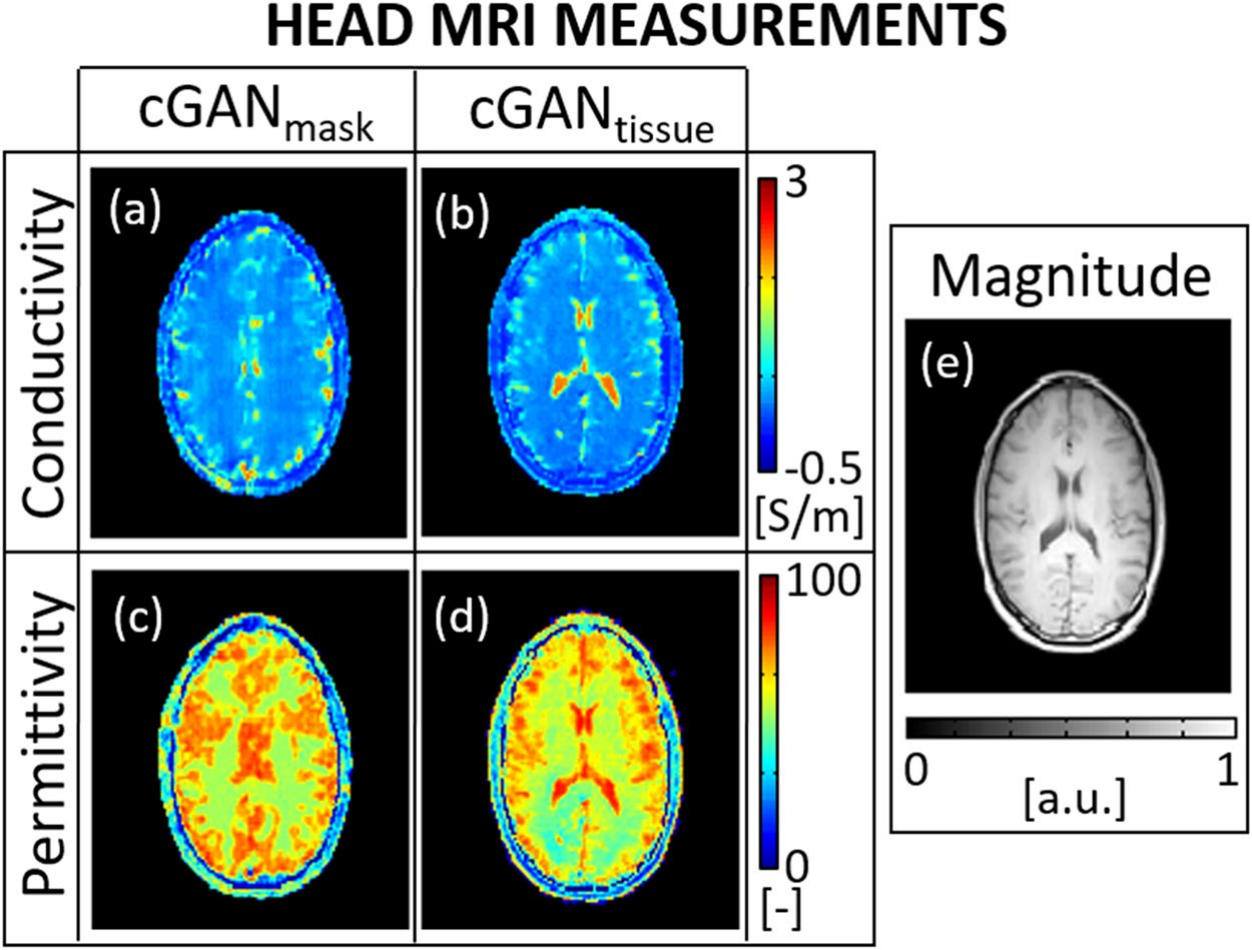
DL-EPT conductivity and permittivity reconstructions from MR measurements on the first subject using cGAN_mask_ and cGAN_tissue_ (a–d). The correspondent MRI magnitude image is also shown as a reference (e).
